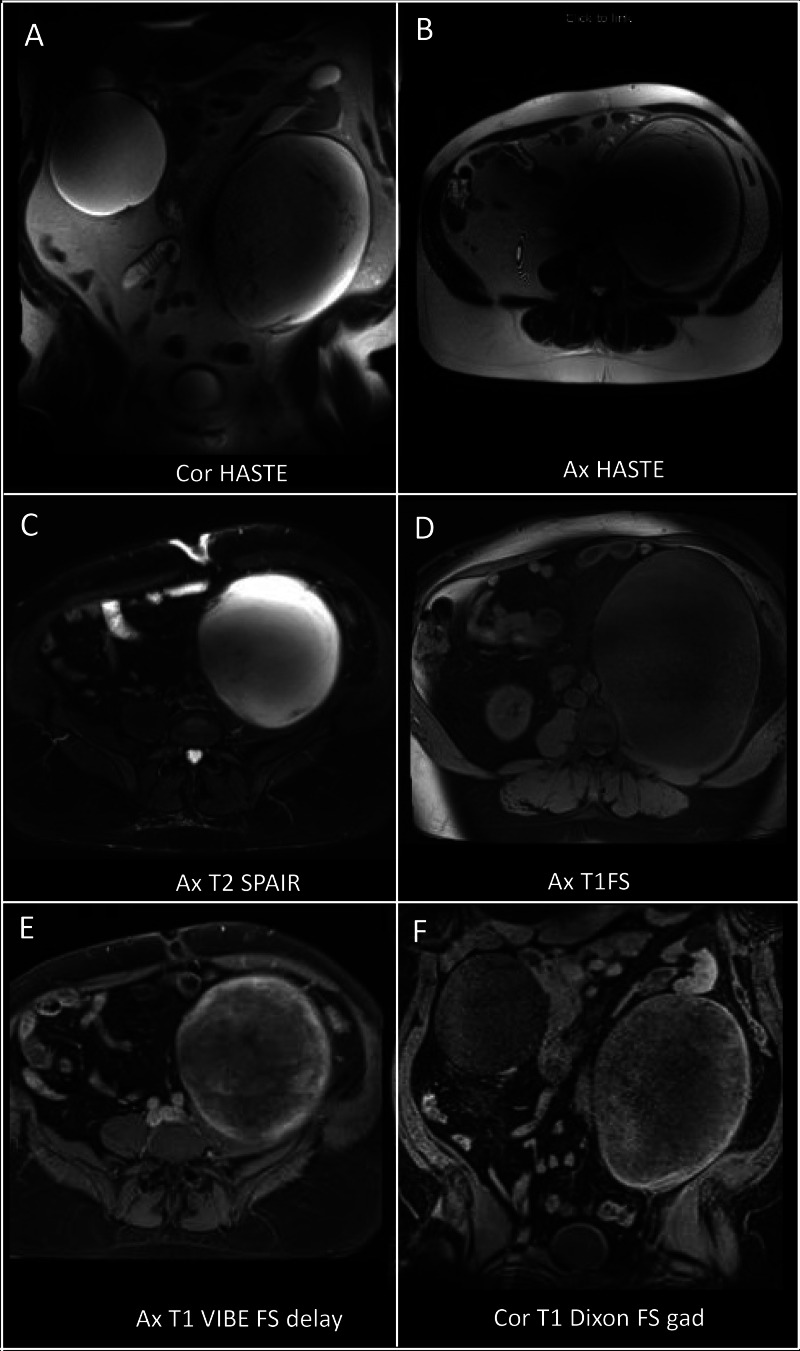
Multisequential and multiplanar MRI of the abdomen and pelvis shows a predominantly T2 hyperintense and T1 hypointense lesion (A–D). The peripheral aspect of the lesion exhibits mild enhancement (E, F). No perilesional edema is noted. No macroscopic fat component.
